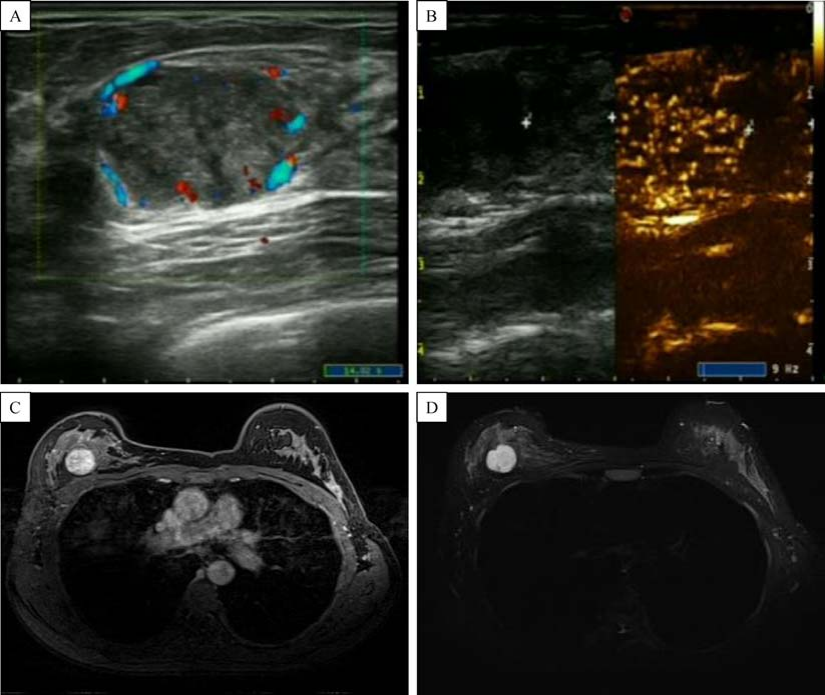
The feture of atypical fibroadenoma. A: The morphology acquired from conventional ultrasound was irregular, with nonhomogeneousinternal echo; peripheral blood flow could be seen, with internal blood flow slightly increased; B: Contrast-enhanced ultrasound was homogeneous, with high enhancement and a clear boundary; C-D: Internal enhancement was non-homogeneous, showing high enhancement. Pathology indicated a fibroadenoma.
